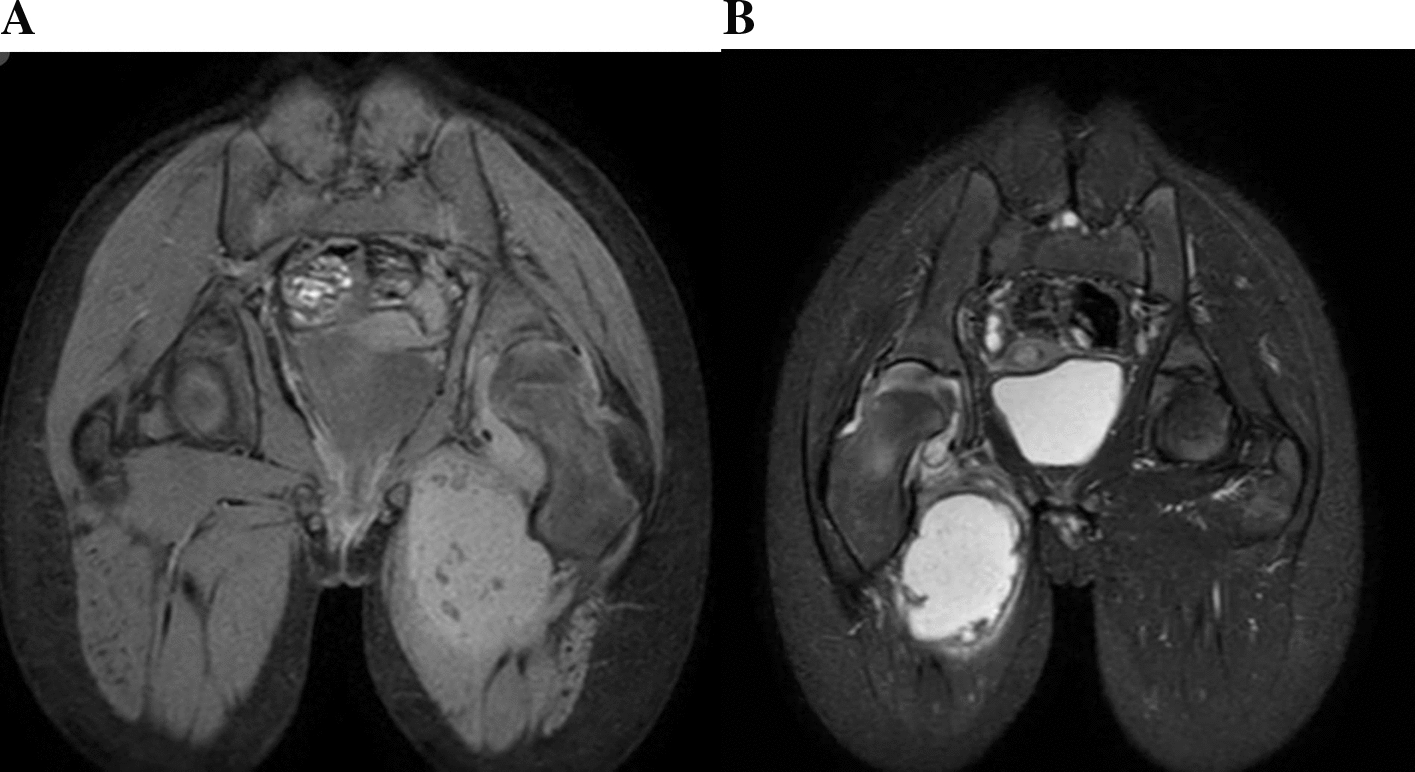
A and B Axial magnetic resonance imaging of the pelvis demonstrates a fluid collection of the fluid in the adductor region, appearing hyperintense on T2-weighted imaging and hypointense on T1, consistent with an abscess. The collection is seen communicating with the left hip joint space, raising concern for septic arthritis. Adjacent soft tissue edema is also noted, without evidence of osteomyelitis
